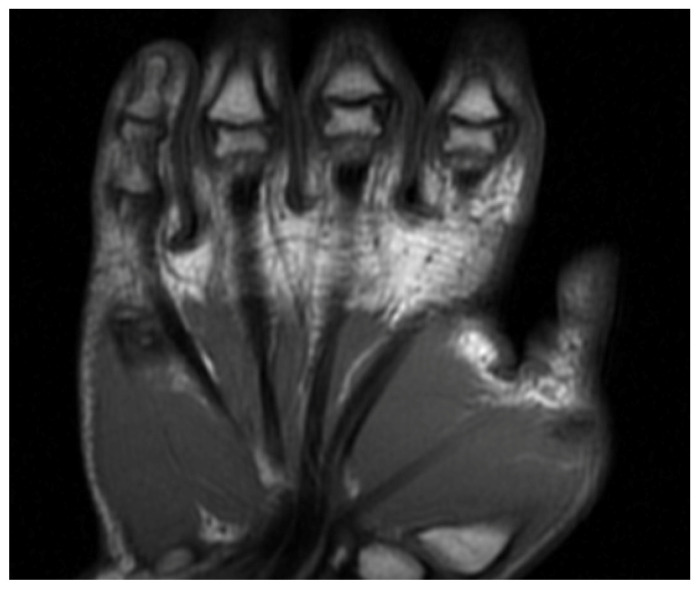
MRI of the hand—mild T2 hyperintensity of periarticular soft tissues; no tendinitis.
